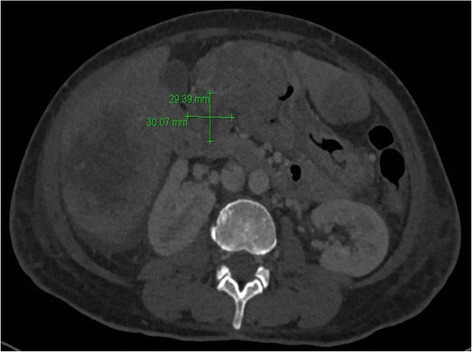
Adenocarcinoma involving the antrum of the stomach. Computed tomographic scan of the abdomen and pelvis with intravenous contrast shows an ill-defined 3cm×2.9cm necrotic mass. Scan also reveals omental implants and nodular extension of the gastric tumor posteriorly into the lesser sac
